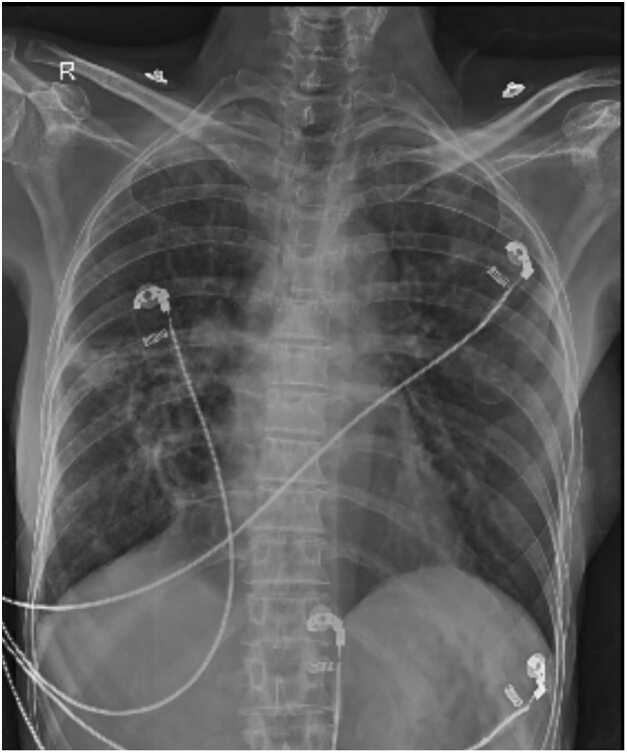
Follow-up Chest X-ray (PA view). Image obtained 18 months post-treatment shows marked resolution of the previous right middle and lower zone opacity, with residual fibrotic changes.
